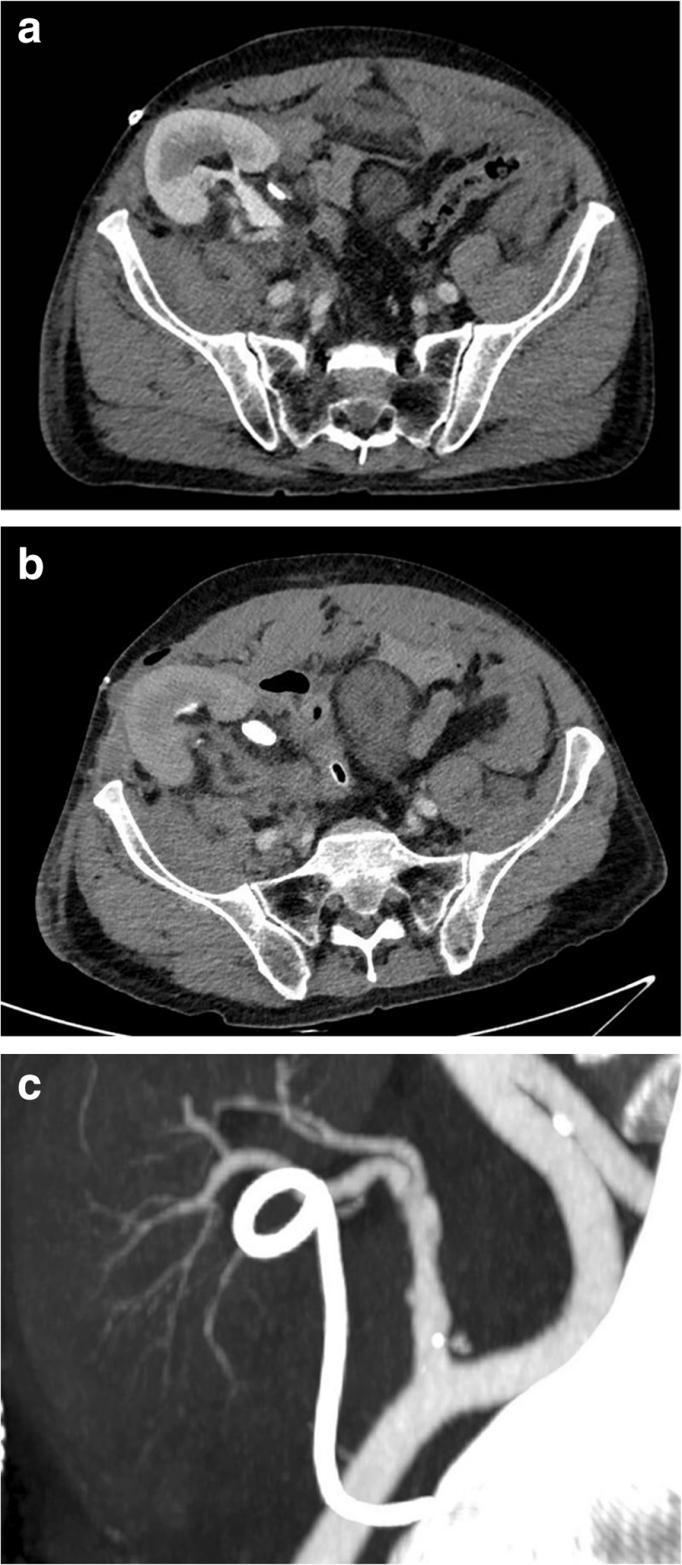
TC scan at different time: a at the time of patient pain, b few hours after the first CT scan and c revision of the imagining by an expert radiologist
